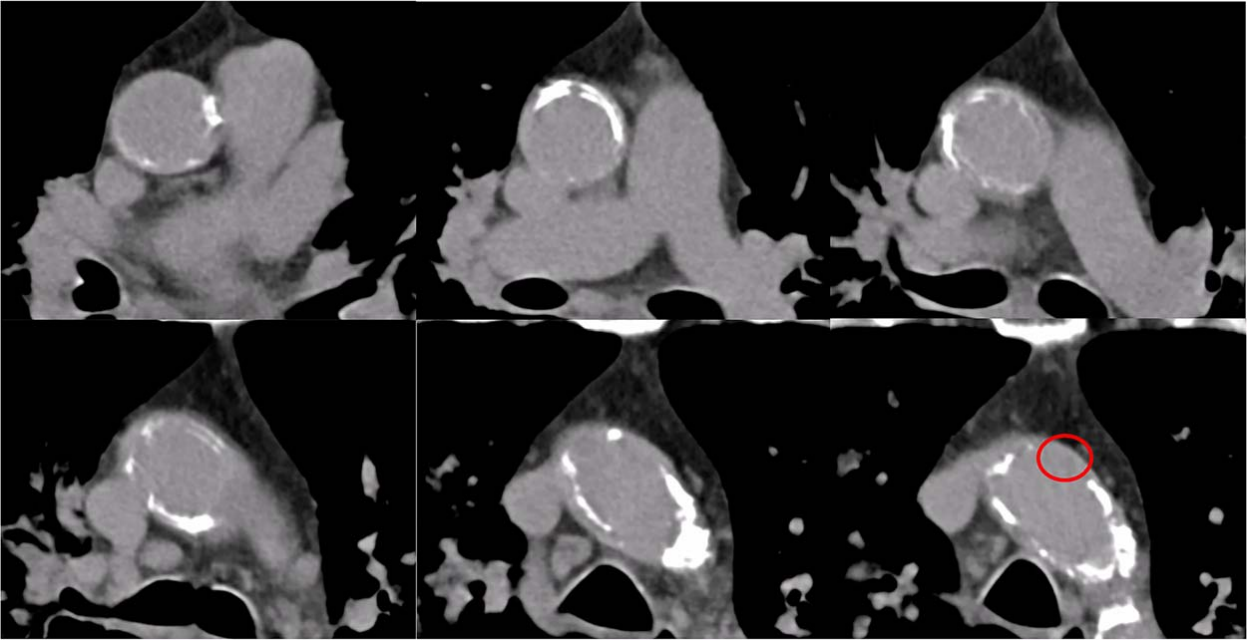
Preoperative contrast-enhanced CT of the ascending aorta. The ascending aorta is diffusely calcified. A region near the origin of the brachiocephalic artery is considered suitable for arterial cannulation. A proximal site above the fat band is also identified as appropriate for SVG anastomosis.
